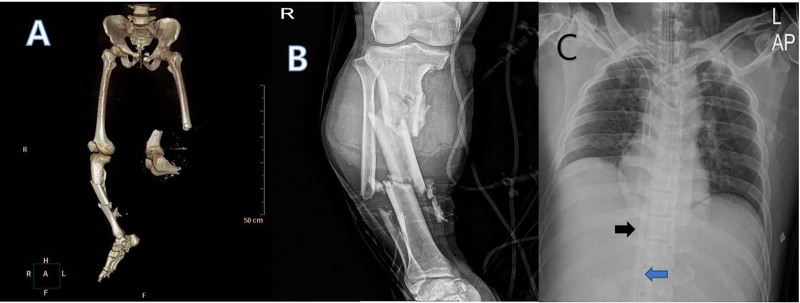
Three-dimensional computed tomography and simple radiography of legs shows amputation state of the left lower extremity (A) and fracture of the right tibia, right fibula, and right calcaneus (B). Veno-venous extracorporeal membrane oxygenation was applied peripherally through both femoral veins using a 21 (black arrow), 23 Fr (blue arrow) venous cannula (C).
